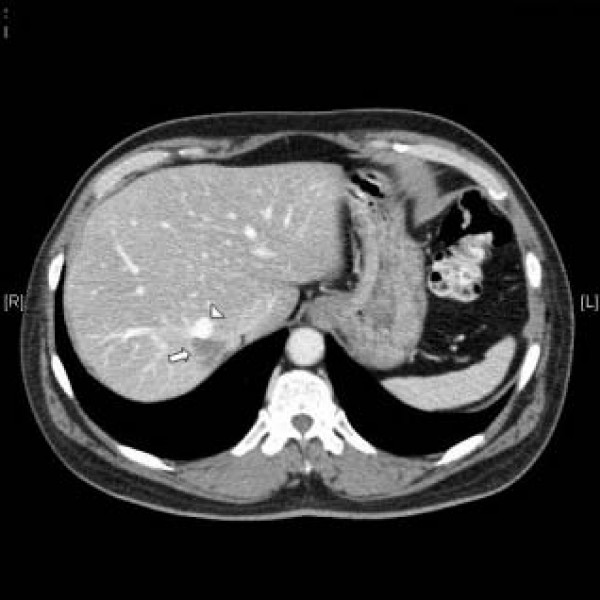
Transversal CT image of the patient's liver showing the right (arrowhead) in proximity to the tumor (arrow).
